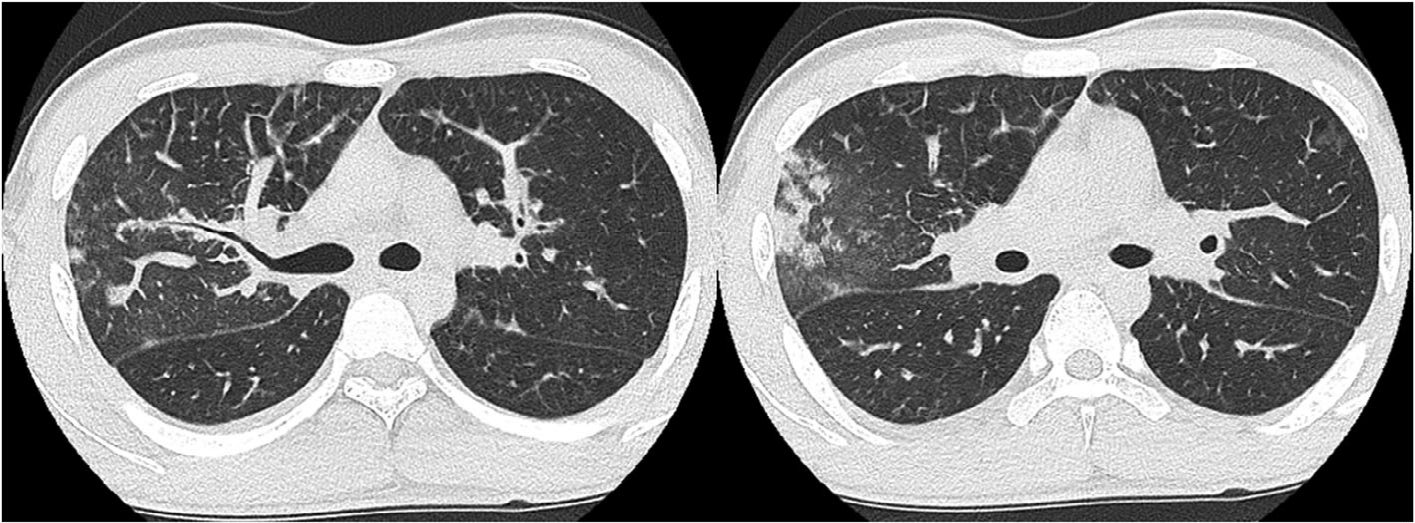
A chest computed tomography scan in the emergency department showed thickening of the bronchovascular bundles and interlobular septal walls in both lungs. In particular, the right upper lobe showed a granular shadow, nodules and ground‐glass opacity under the pleura. A small amount of pleural fluid was observed on the right side.
